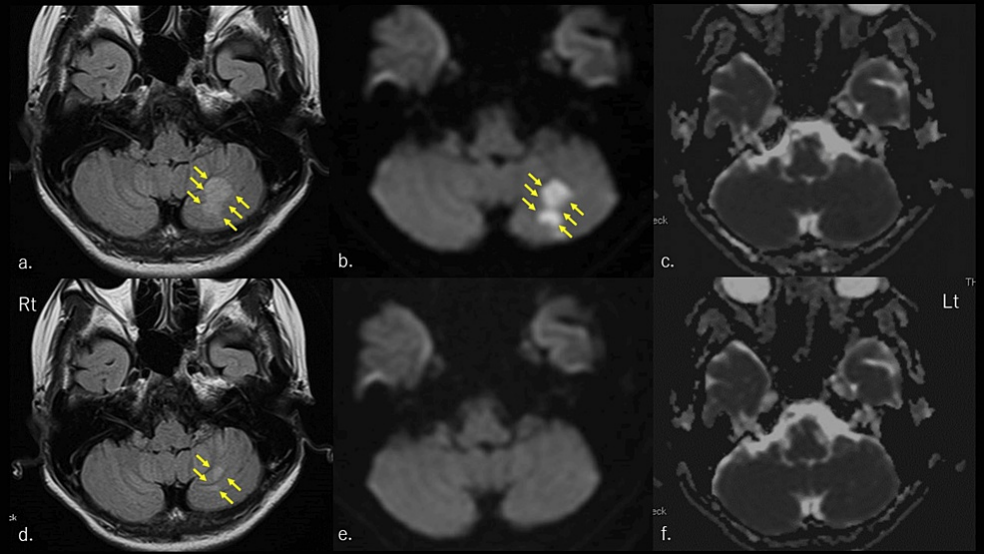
Head magnetic resonance imaging. The upper-row images are taken during the initial presentation. Arrows point to hyperintensities associated with PRES. (a) FLAIR sequences showing hyperintensities in the left cerebellar regions. (b) DWI showing hyperintensities in the left cerebellar regions. We considered it is T2 shine through. (c) ADC maps show a normal appearance in the left cerebellar regions. The lower-row images were taken in the third week after disease onset. (d) FLAIR sequences showing slight hyperintensities in the left cerebellar regions. (e, f) DWI and ADC maps showing normal appearance in the left cerebellar regions. PRES: posterior reversible encephalopathy syndrome; FLAIR: fluid-attenuated inversion recovery; DWI: diffusion-weighted imaging, ADC: apparent diffusion coefficient
